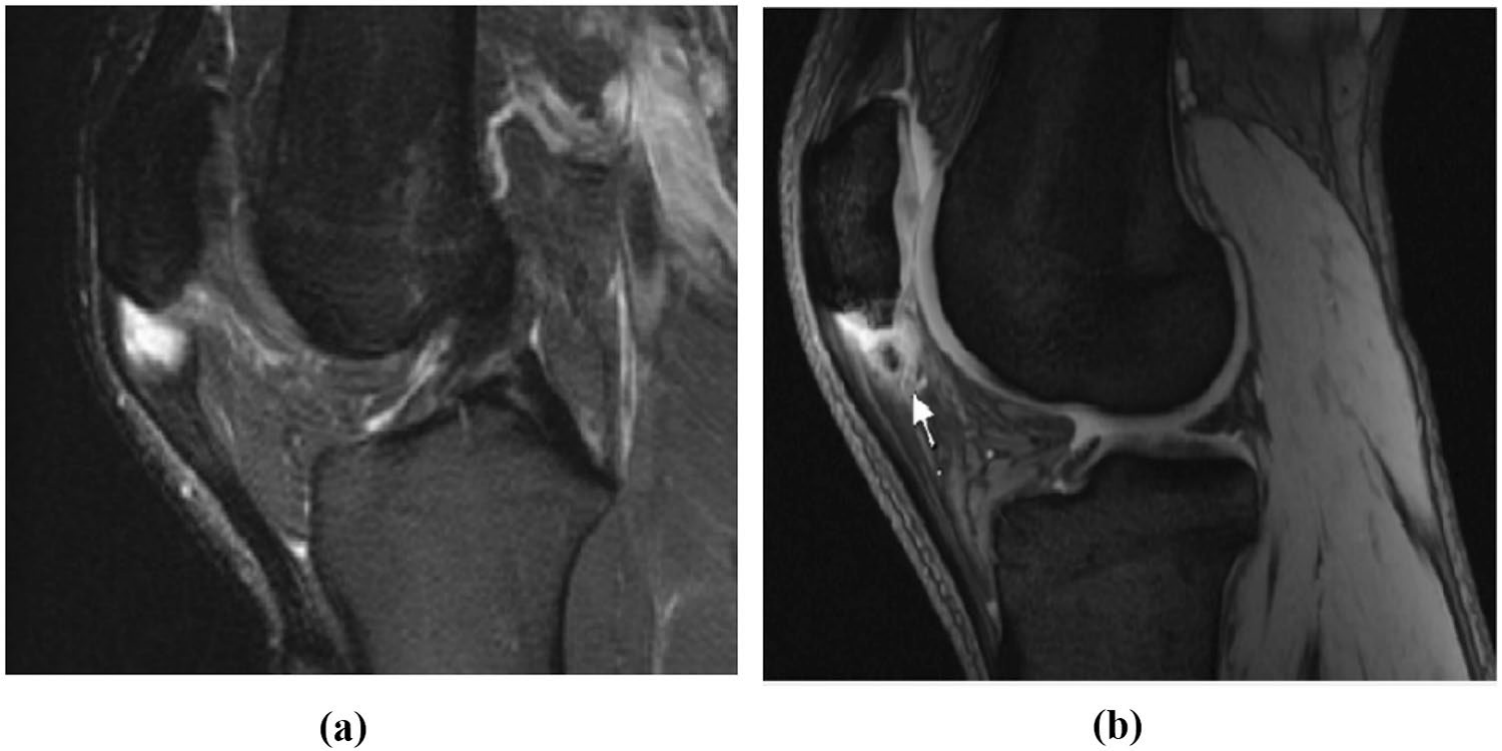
a T2-weighted MRI image of the left knee, in a young elite athlete with PT symptoms. It demonstrates an increased signal interposed in the patellar tendon. b T2-weighted MRI image of the left knee, in a young elite athlete with PT symptoms, showing a free bone formation in the mass of the patellar tendon
